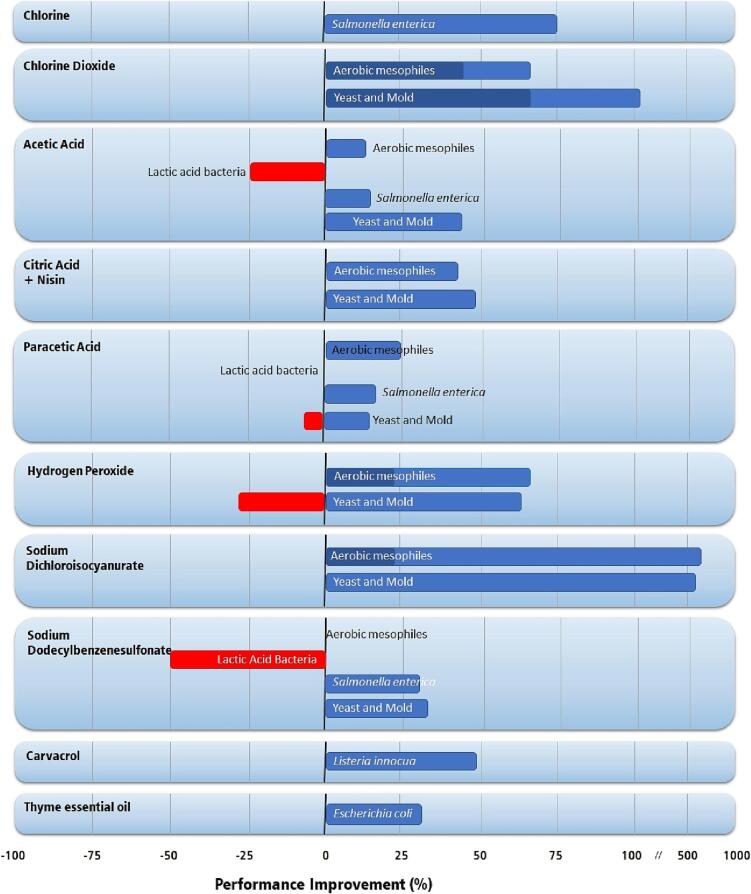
Improvement in the performance of sanitizers by ultrasound application during the initial washing and sanitizing stage of fruits and vegetables. Data were obtained in the literature [61], [63], [64], [65], [68], [69], [71], [55]. For case studies reported by more than one article, dark blue and red bars indicate the minimal values reported for performance improvement.
